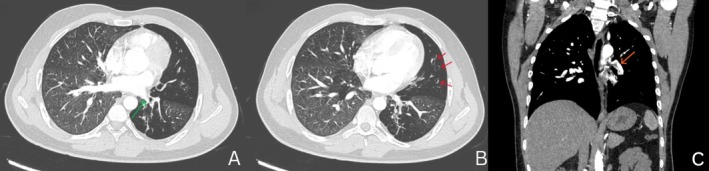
Computed tomography (CT) of the chest (A–C). (A) and (B) axial high‐resolution CT images showing diffuse hyperlucent areas in the left lung, along with fibrotic and bronchiectatic changes (red arrows) in the lower lobe and lingula, reduced left lung volume, and a hypoplastic left pulmonary artery (green arrow)—features suggestive of Swyer‐James‐MacLeod syndrome. (C) Coronal CT pulmonary angiogram image showing hypertrophied left bronchial artery (orange arrow).
